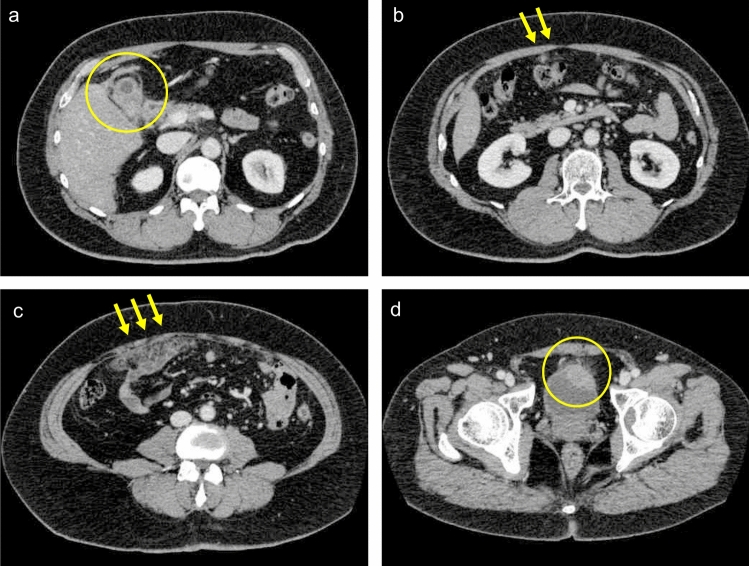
Contrast-enhanced abdominal CT findings. a Irregular wall thickening of the gallbladder. b Multiple peritoneal nodules. c Omental thickening. d A urinary bladder tumor
